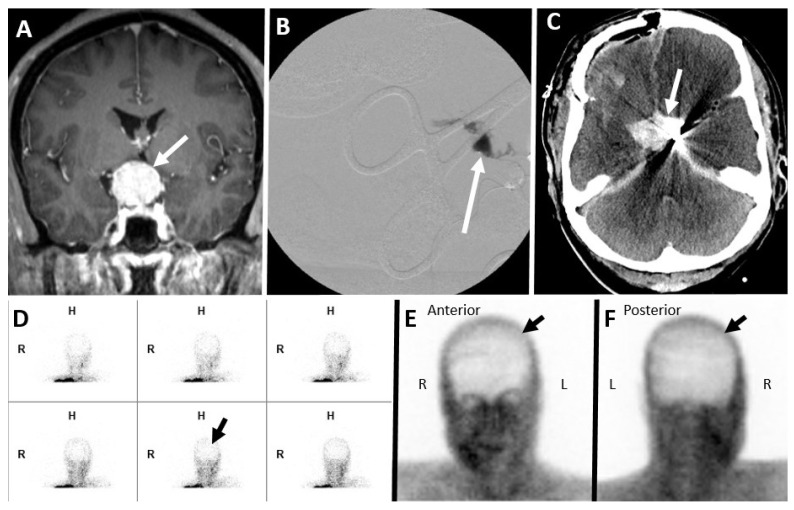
Forty-nine-year-old female with suprasellar mass lesion. (A) Coronal T1 post contrast image shows enhancing suprasellar mass lesion (white arrow in (A)). Patient underwent surgical resection and developed intra-operative massive bleeding. (B) Intra-operative fluoroscopy image shows contrast extravasation from avulsed cavernous left internal carotid artery, and the patient underwent embolization and repair for the same issue. (C) Axial unenhanced CT image of the brain shows hematoma in the basal cistern (white arrow) with streak artifact from the coil embolization of left internal carotid artery with post-surgical change. Tc-99m DTPA nuclear scintigraphy study, (D) initial blood flow phase images, (E) static anterior, and (F) posterior images demonstrate the absence of intracranial tracer activity (black arrows in (D–F)), confirming BD/DNC. There is tracer activity in the right scalp from recent surgery.
